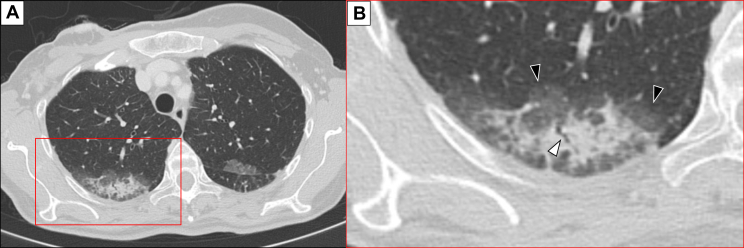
Same patient as in Figure 1, who experienced interstitial lung disease induced by trastuzumab deruxtecan therapy. (A) Lung consolidation (red square in A) seen in the right upper lobe of the non-contrast computed tomography scan carried out in August 2022. (B) Detail of the same lung consolidation, in which it is possible to observe the presence of air bronchogram within the consolidation (white arrowhead) and ground glass opacities (black arrowheads) around it.
